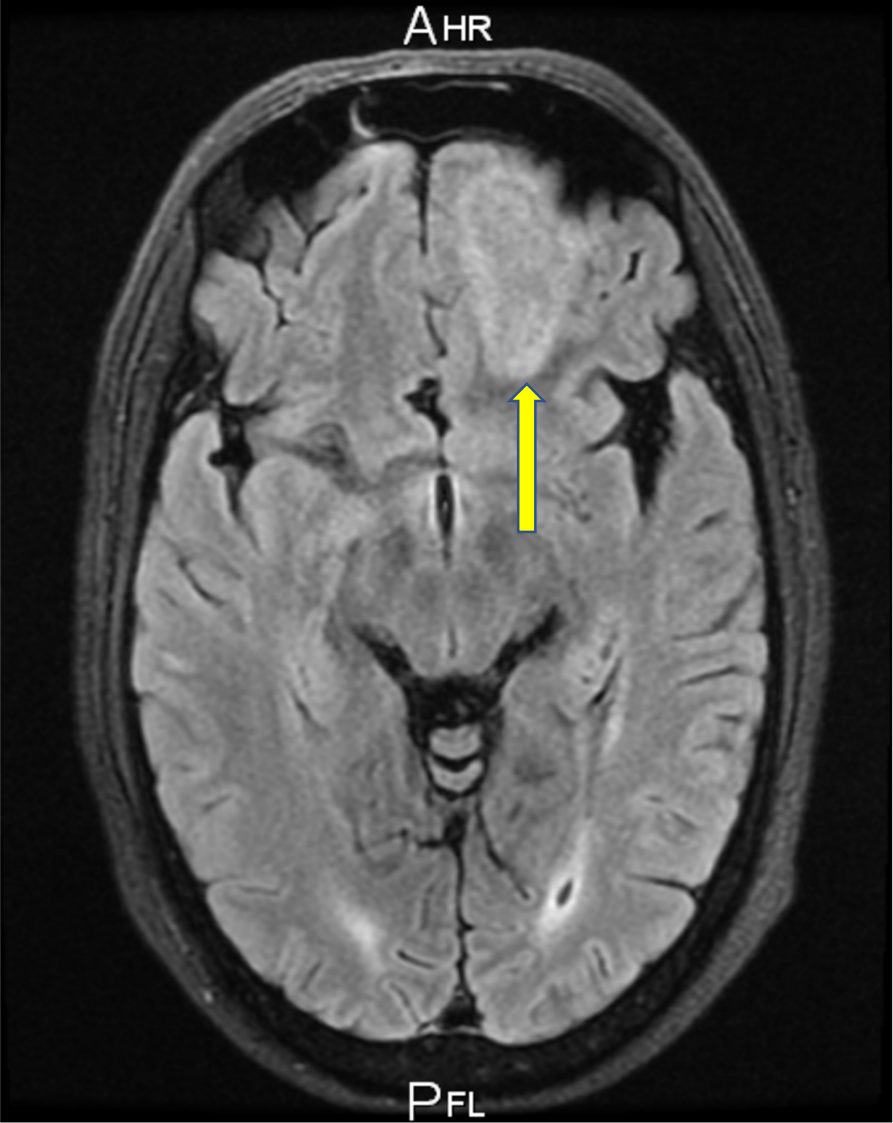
MRI scan of the brain with contrast T2 FLAIR sequence-frontal abscess
